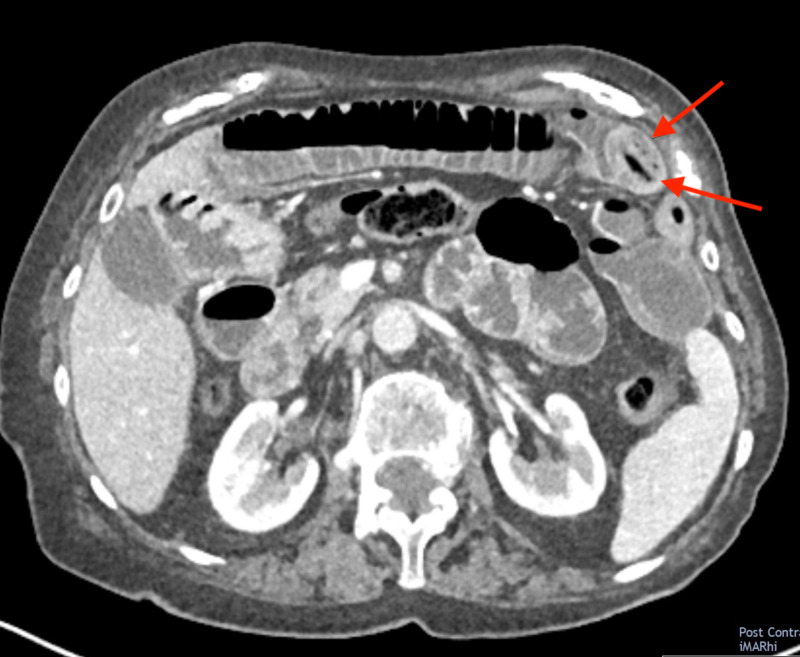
Axial CT image showing an obstructing enterolith (arrows) that has likely migrated from its original location in the inflamed jejunal diverticulum (see Figure 1) and now appears lodged in a loop of mid-small bowel.
